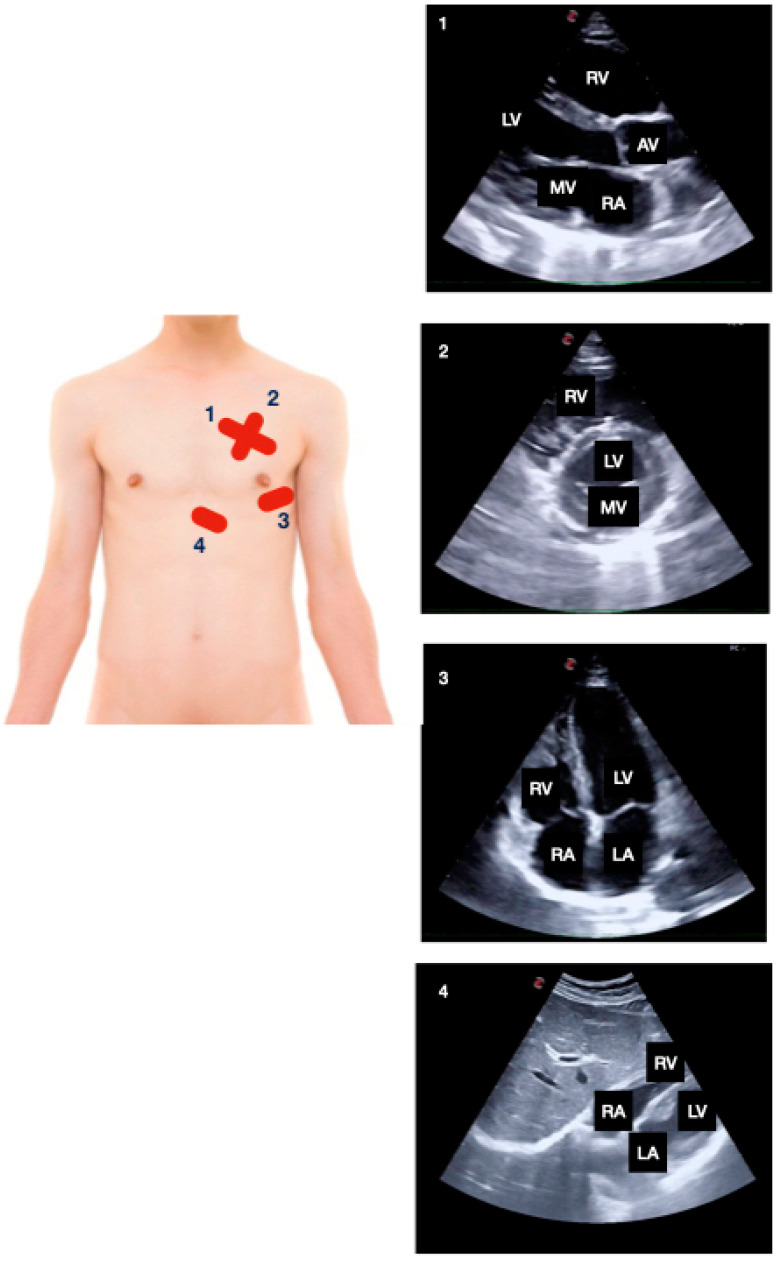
Focused cardiac ultrasound: (1) parasternal long and (2) short axis, (3) apical four chambers and (4) subxiphoid. AV: aortic valve, LA: left atrium, LV: left ventricle, MV: mitral valve, RA: right atrium, RV: right ventricle.
